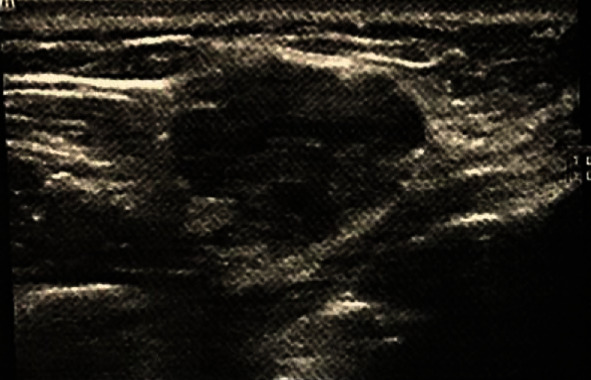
Ultrasonography shows a well-defined hypoechoic heterogenous mass lying between the skin and buccinator muscle.
